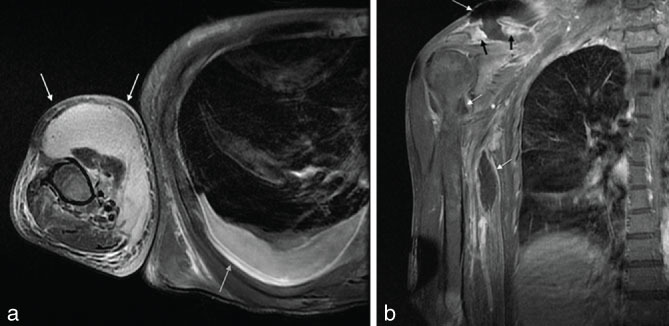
MRI of the right shoulder. (a) *T*
